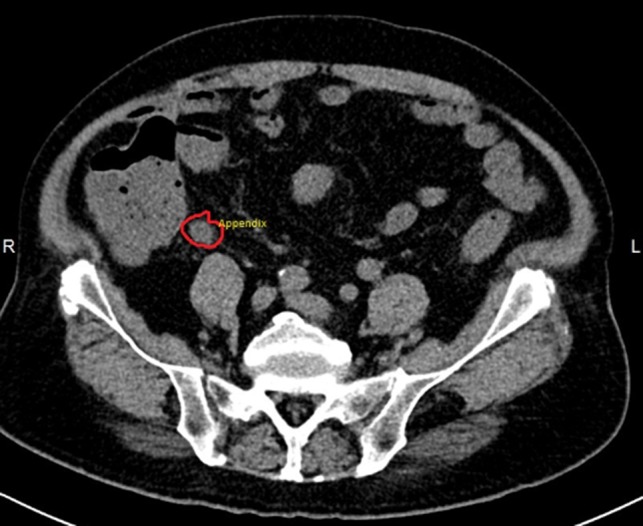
CT aortogram image showing the swollen appendix
